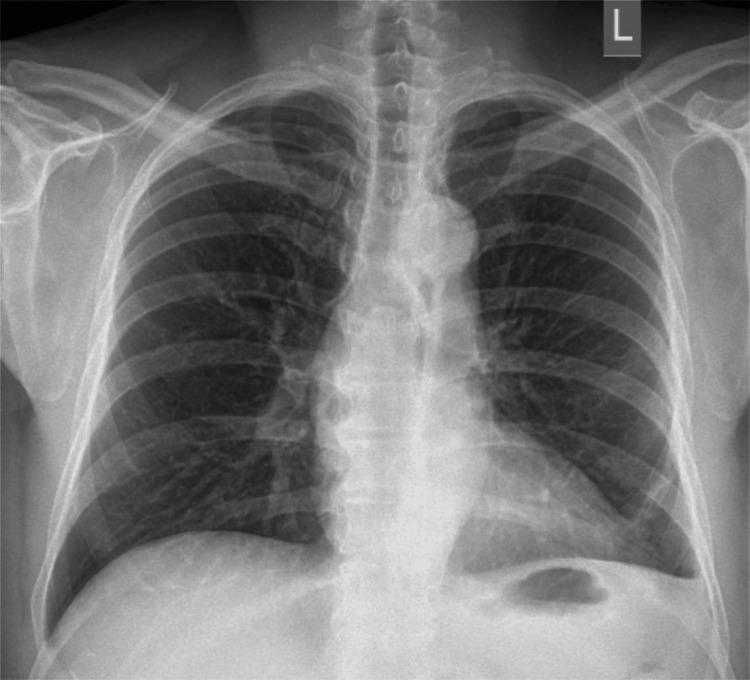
Chest X-ray after four weeks of ATT showing complete resolution of the effusion. ATT: Antitubercular Treatment.
